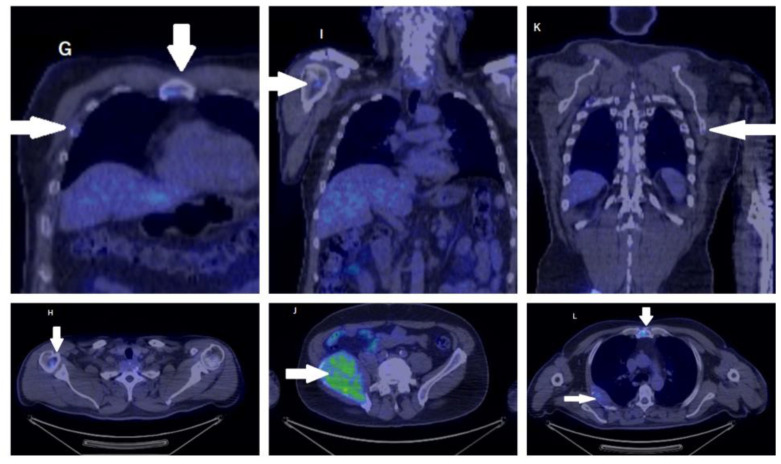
PET-CT showed an activated metabolism (arrow) of a mass in the right iliac wing of 10/7.5 cm that invaded the neighboring endo- and exopelvic structures. The mass showed an inhomogeneous uptake of FDG. Other similarly moderate FDG-capturing osteolytic lesions could be distinguished in the right posterior fourth costal arch (invasive and dimensional progression compared to the CT scan performed 1 week before), the right fourth anterior costal arch, the left sixth lateral costal arch, the sternal manubrium, the medial angle of the scapula on the left, the C7 (with a major risk of subsidence), the T7 and T9 vertebral bodies, the right lateral clavicular extremity, the right humeral head, the apex, the right temporal bone, right parietal bone, and the left sciatic tuberosity.
